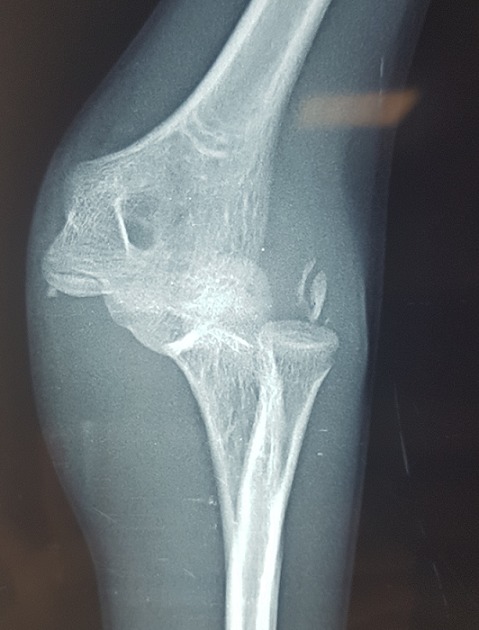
Luxation du coude associée à une fracture de l’épicondyle médial et latéral sur une radiographie de face
